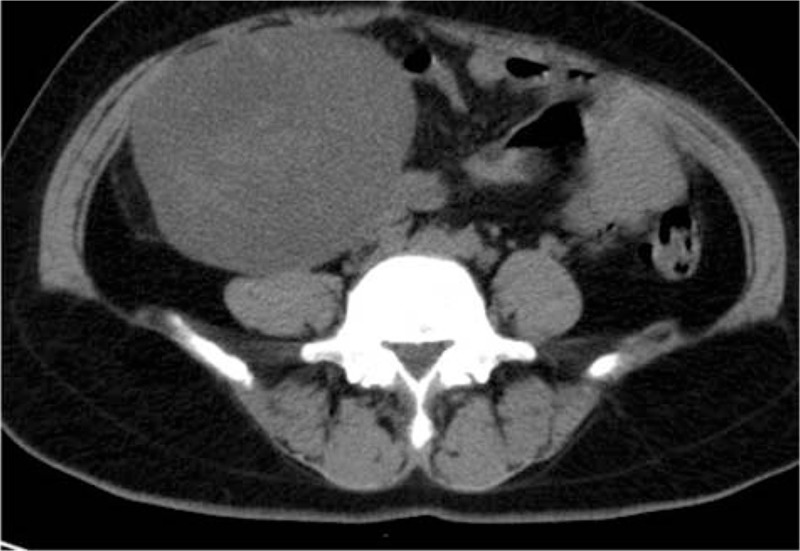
A pelvic mass examined by computed tomography (CT). CT examination revealed a well-defined mass in the posterior uterine region, which was considered fibroid steatosis.
